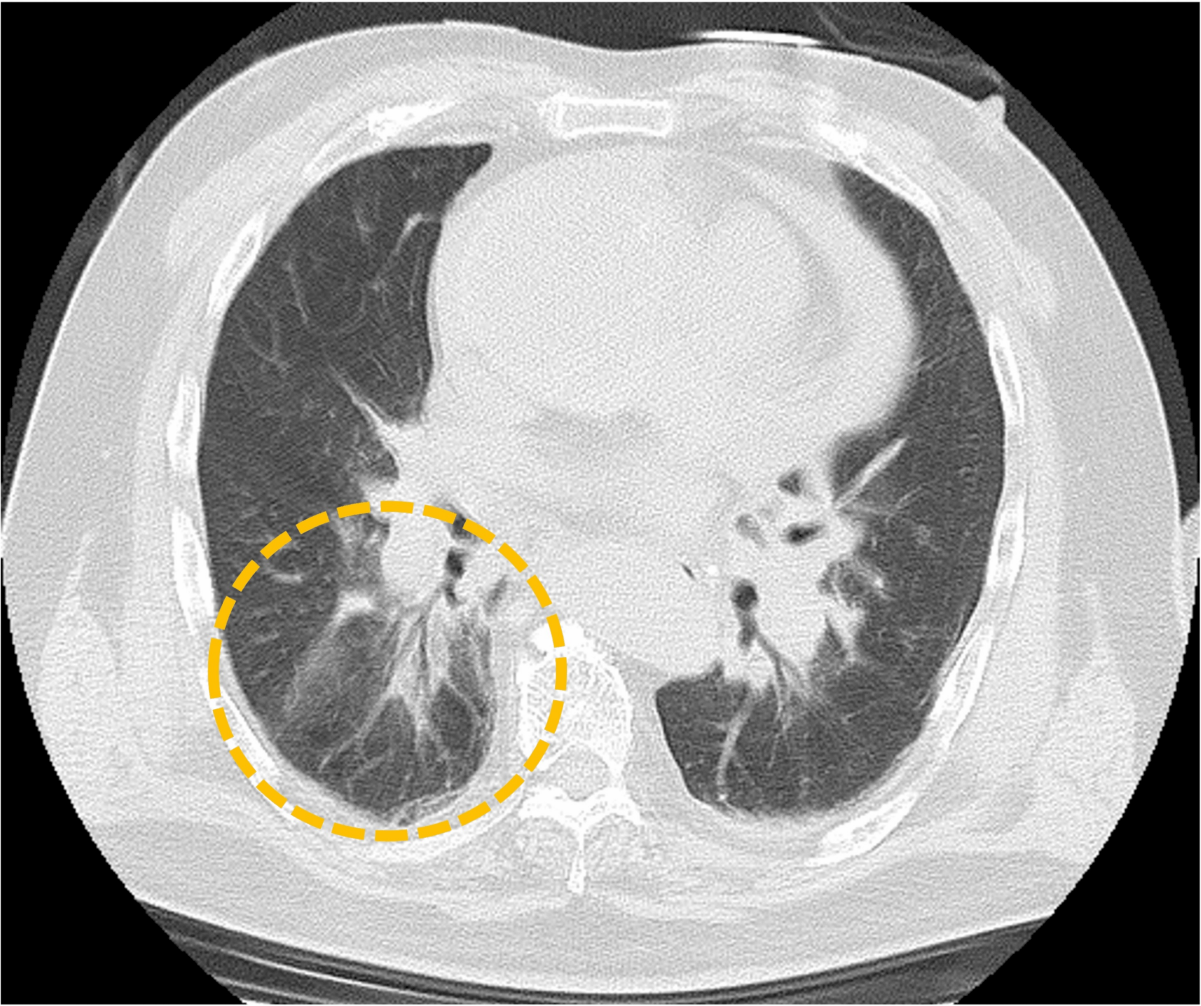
Non-contrast computed tomography in lung window settings In lung window settings, a ground-glass opacity is observed surrounding the hematoma in the right lower lobe pulmonary artery branch, suggesting alveolar hemorrhage (yellow circle).
